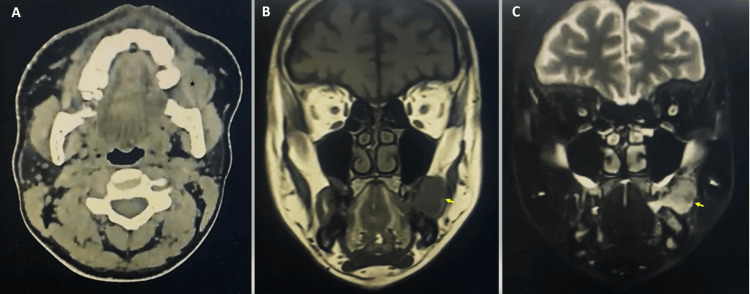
Radiographic imaging A) CT scan showed a soft tissue density mass in the left cheek (asterisk). B) MRI showed a well-circumscribed mass, hypointense in T1. C) Iso to hyperintense mass in T2 (arrows).
